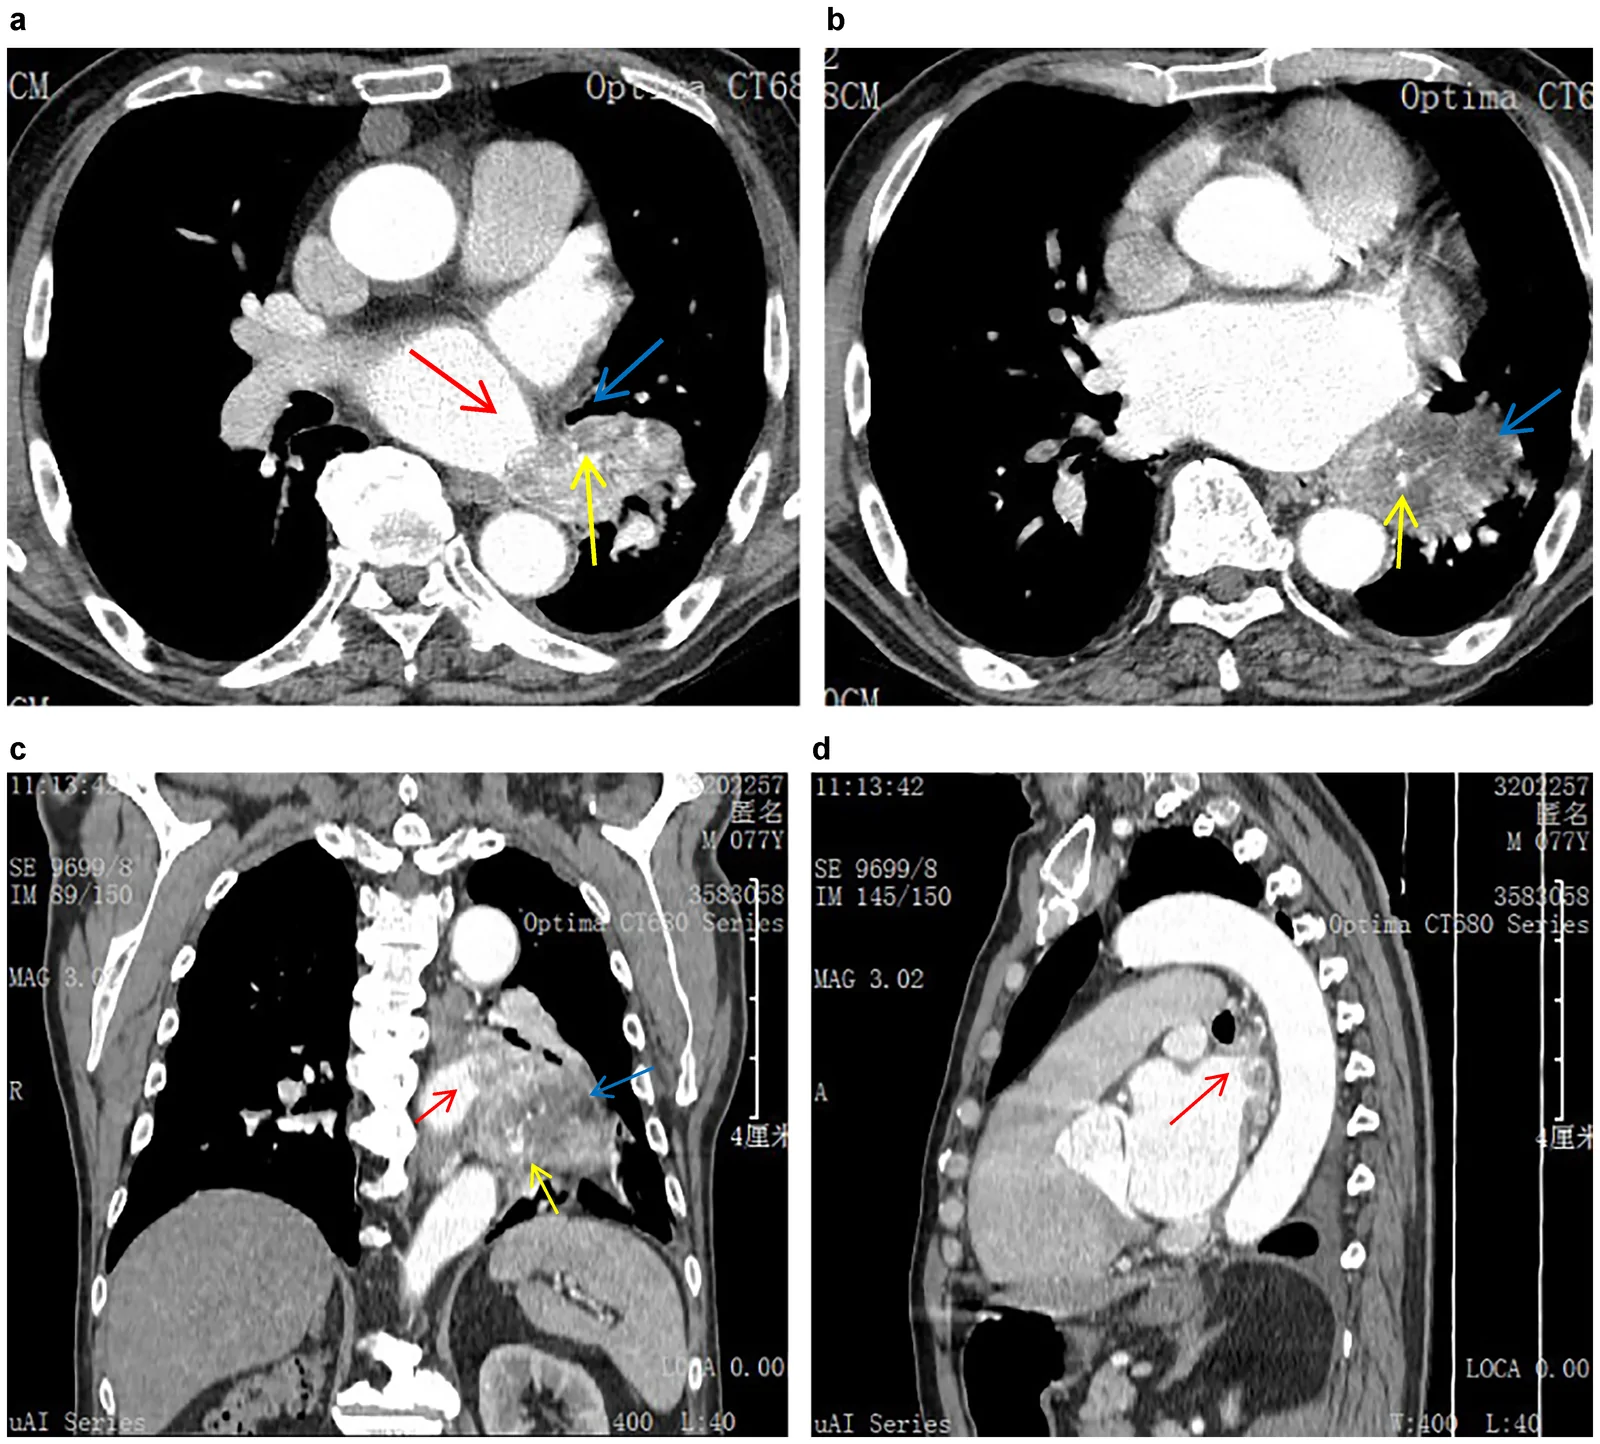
Chest contrast-enhanced computed tomography (CT). (a, b) Axially enhanced chest CT scan. (c) Coronal. (d) Sagittal. Mass-like soft tissue shadow (approximately 70 × 45 mm) was present adjacent to the mediastinum in the lower lobe of the left lung, with unclear boundaries and low-density areas (blue arrow). The average CT value of the lesion was approximately 38 HU, while the low-density region measured approximately 19 HU. The lesion demonstrated heterogeneous enhancement with CT values of approximately 130 HU in the region of marked enhancement and 23 HU in the low-density area. The lesion showed tortuous traversing vascular (yellow arrow) shadowed invading the left pulmonary vein (red arrow).
